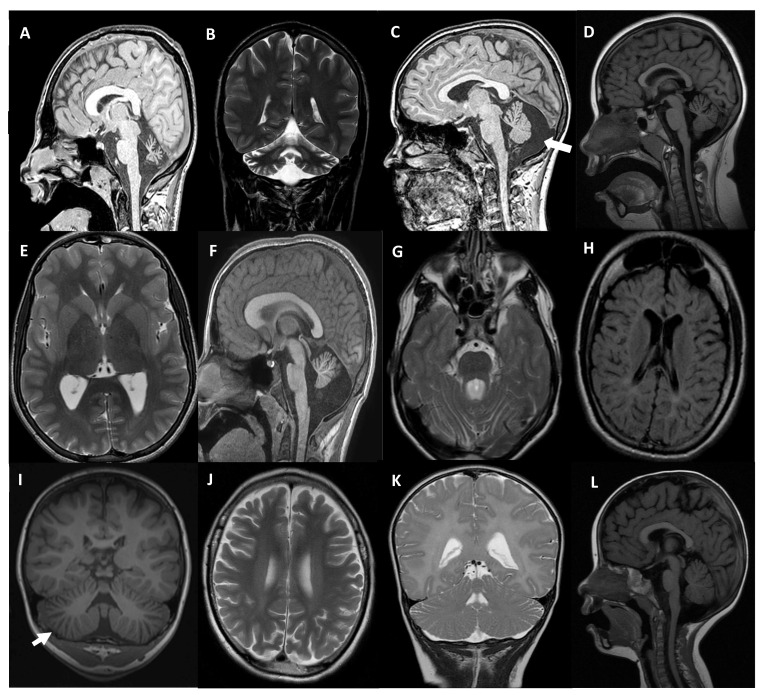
Main neuroimaging signs. (A,B) MD-353/*CACNA1G*, severe vermian atrophy in mid-sagittal T1-weighted image (A) and mild global atrophy in both cerebellar hemispheres in coronal T2 (B) at 17 years of age. (C) MD-309/*CACNA1A*, a 12 year-old with moderate atrophy in the anterior lobe of the cerebellar vermis and a mega cisterna magna in mid-sagittal T1 (white arrow). (D) MD-556/*CACNA1A* at 10 years, moderate atrophy in the anterior lobe of the cerebellar vermis (T1-weighted sagittal image). (E,F) MD-548/*MAST1* at 13 years, T2 axial image showing thinning of anterior arm of internal capsule (E), and T1 mid-sagittal image with a globally increased corpus callosum and vermian reduced volume (F). (G) MD-392/*CPLANE1* at 17 years, T2 axial image showing a lack of normal decussation of superior cerebellar peduncular fiber tracts that follow and horizontal course (molar tooth sign). (H) MD-297/*TBCD* at 18 years, T2 FLAIR axial image revealing a striking reduction of supratentorial white matter, severe brainstem and cerebellar atrophy was also ascertained. (I,J) MD-471/*CLN6*, at 5 years (I), T1 coronal image showing enlarged cerebellar folia spaces (white arrow) at 6.5 years (J), T2 axial image disclosed cortical atrophy and deep/periventricular white matter hyperintensities. (K) MD-610/*PI4KA*, at 30 months, T2 coronal image showing diffuse hypomyelination and enlarged cerebellar folia interspaces. (L) MD-436/*CLK2* at 20 months of age, with mild enlargement of folia spaces in the anterior lobe of the cerebellar vermis in mid-sagittal T1.
